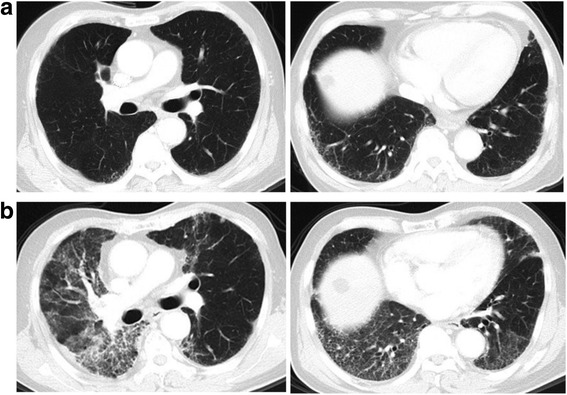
A 75-year-old male with small-cell lung cancer having preexisting radiological ILAs who developed RP beyond radiation field after TRT. a Prior to RT, initial HRCT exhibited subpleural reticular opacities at levels of bilateral lower lobes. b 60 Gy in 30 fractions was delivered to a pulmonary tumor in the right upper lobe with mediastinum and right hilar lymphadenopathy. Three weeks after completing RT, the patient developed diffuse interstitial infiltration and consolidation extending beyond radiation field
